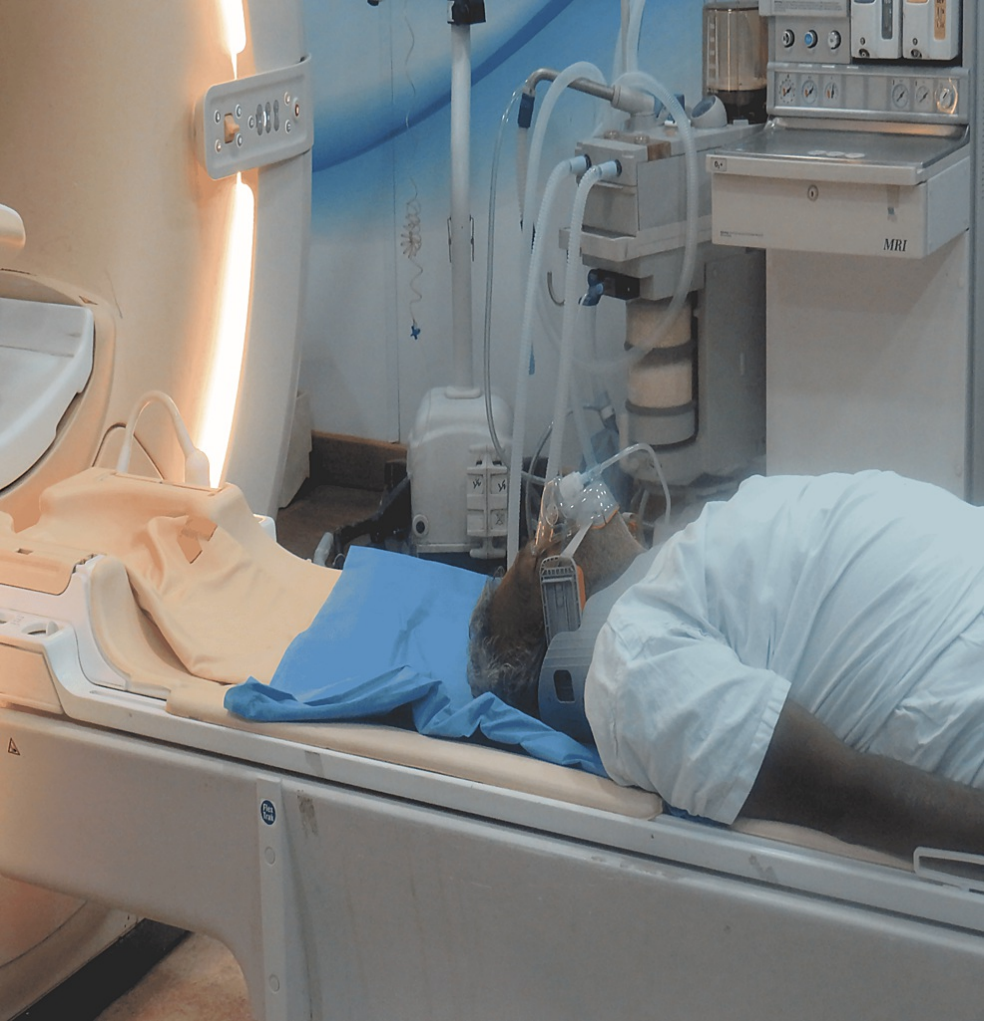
Lubo collar as a rescue during upper airway obstruction in the MRI suite.
